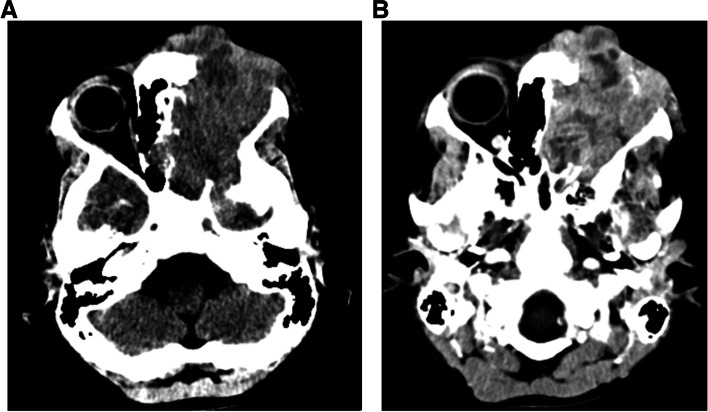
A An unenhanced axial CT at the level of the orbits. There was a large iso-dense mass with in the left orbit, involving the ocular muscles and globe. The mass measured 5.0 × 6.0 cm and had necrotic areas. There was extension to the middle cranial fossa. There were no calcifications seen within the mass. B A contrast enhanced axial CT and demonstrated heterogeneous enhancement of the left orbital mass. A diagnosis of left orbital rhabdomyosarcoma was made on both A and B
